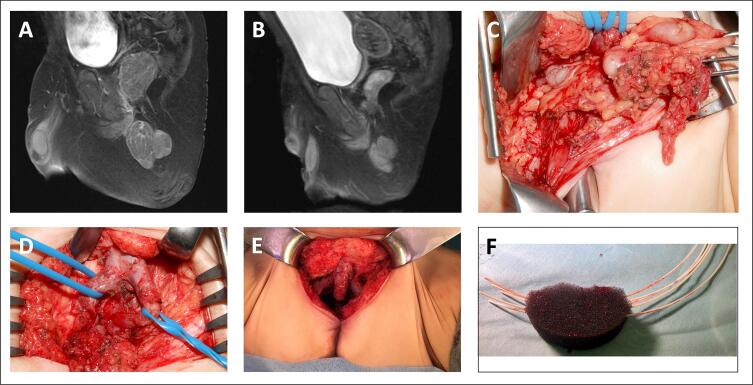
Organ-preserving resection combined with brachytherapy in a 20-month-old boy (patient #4) with embryonal, perianal rhabdomyosarcoma. A) Initial MRI. B) Preoperative MRI. C) Situs before tumor resection. D) Situs after tumor resection with view from perineal. Corpora cavernosa marked by blue loops. E) Situs after tumor resection. F) Sponge with brachytherapy tubes before placement in the wound cavity. (For interpretation of the references to colour in this figure legend, the reader is referred to the web version of this article.)
